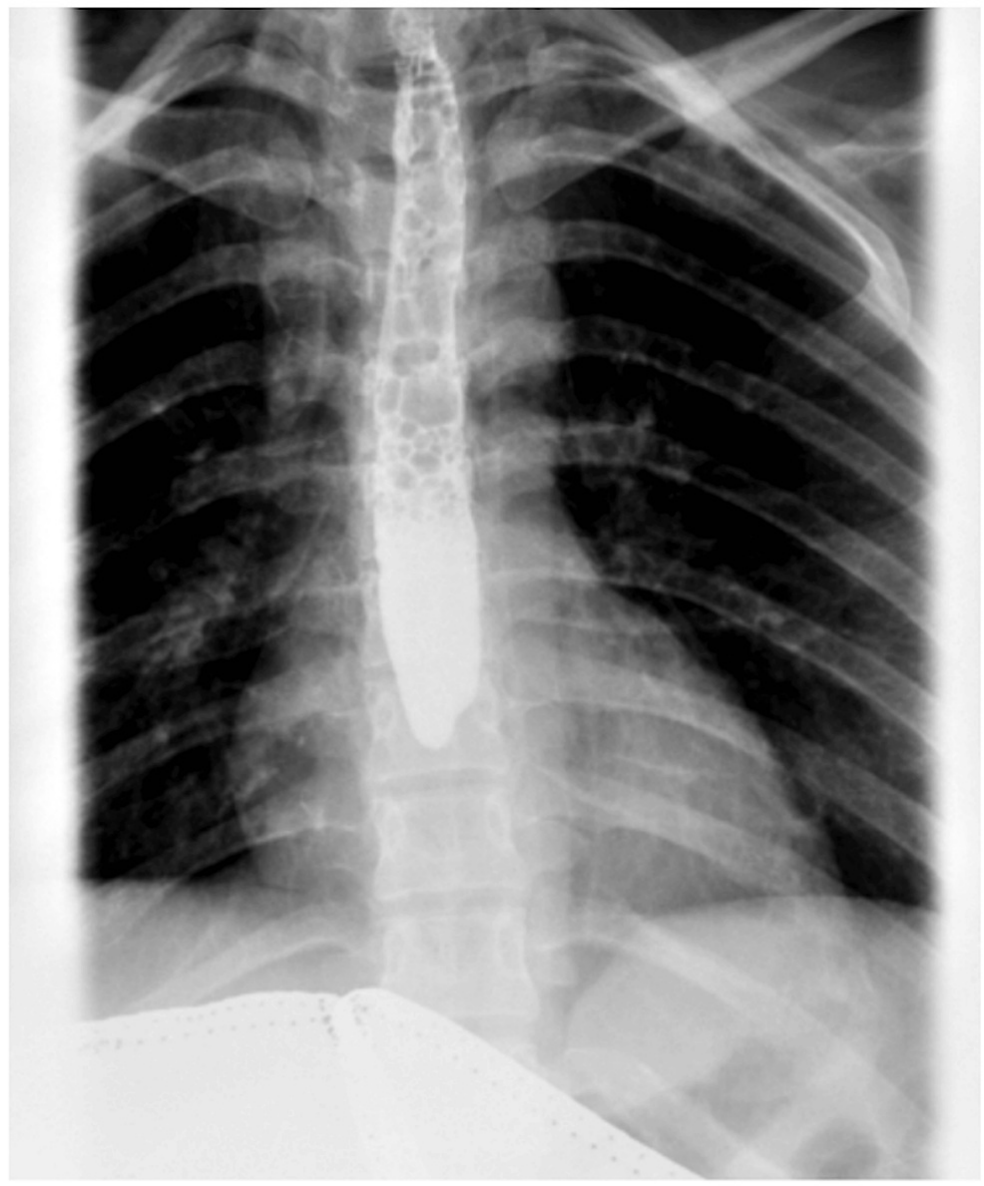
X-ray of the esophagus findings: negative for esophageal tear. Normal in-line flow with peristalsis of the esophagus can be noted.
